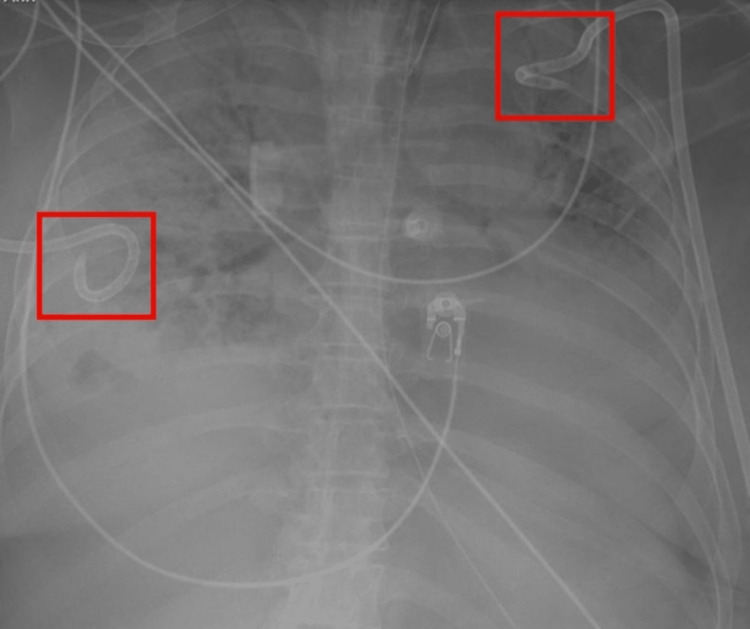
Chest X-ray demonstrating complete opacification of the patient's lungs. The red squares demonstrate the chest tubes.
